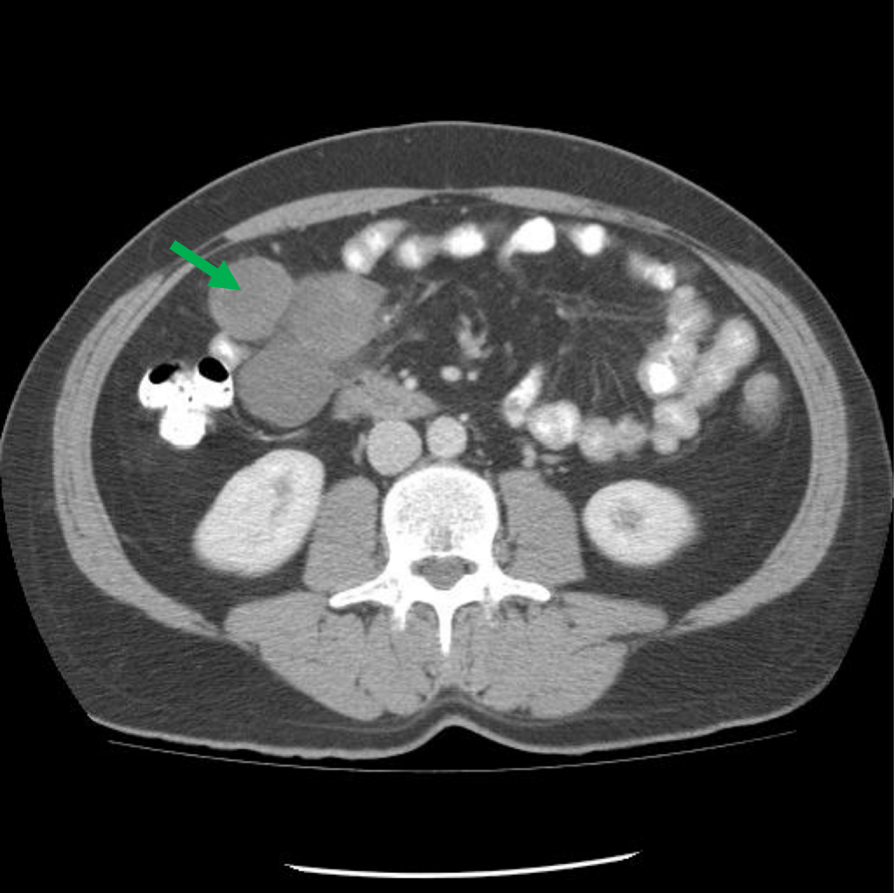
Abdominal CT showed a 10 cm retroperitoneal mesentery mass (put arrows on the mass)
